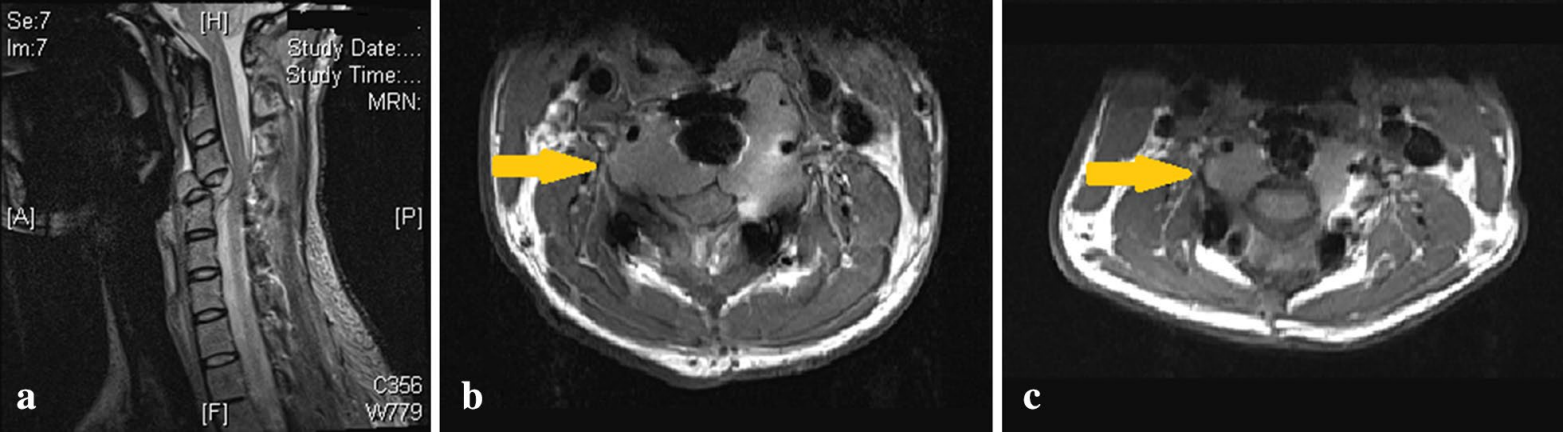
a Acute cervical cord compression secondary to collapse of giant cell tumour in C4 vertebrae. b 4 weeks from emergency decompression and stabilization, patient developed tumour recurrence (*arrow*) causing canal compression. c MRI scan after 3 months of Denosumab showing decrease in tumour mass (*arrow*) and decompression of spinal canal
